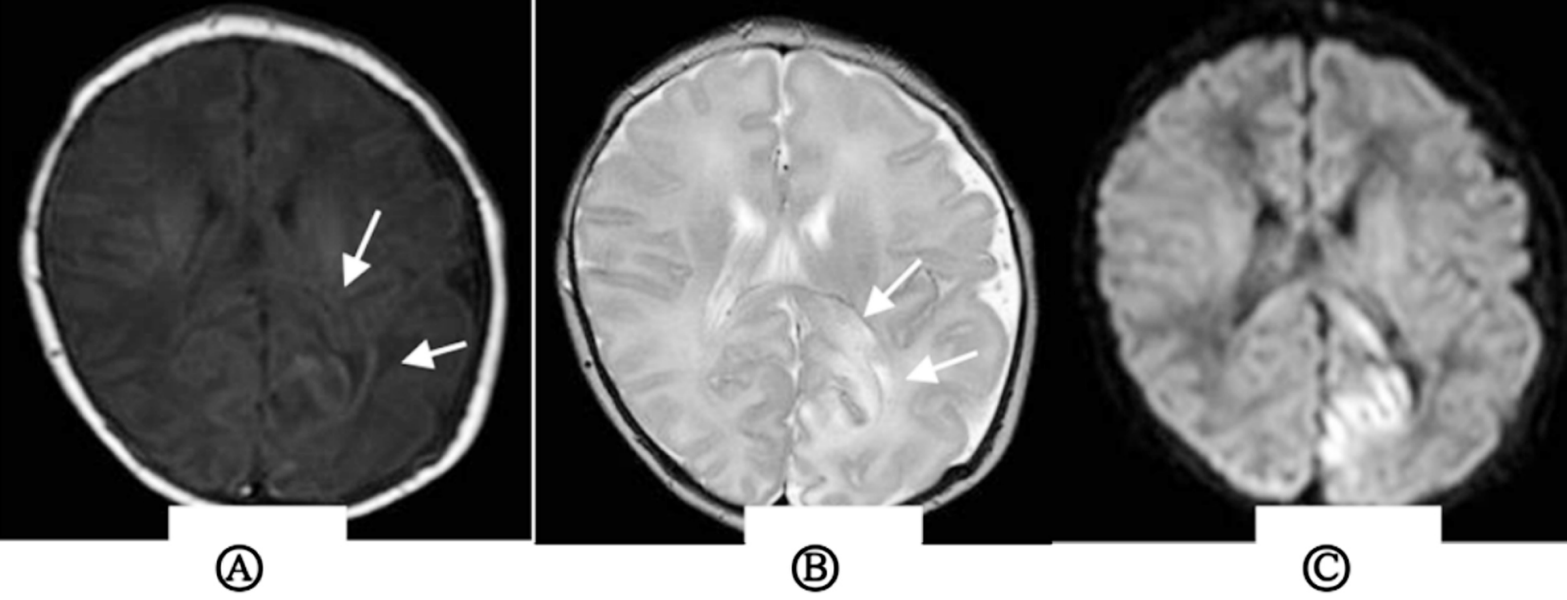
Magnetic resonance imaging findings in the left occipital of neonates with cerebral infarction A) as indicated by the arrows, T1WI suggests a large area of long T1 signal in the left occipital; B) as indicated by the arrow, T2WI suggests a large area of long T2 signal in the lesion area; C) diffusion weighted imaging (DWI) indicates a significant signal in the lesion.
